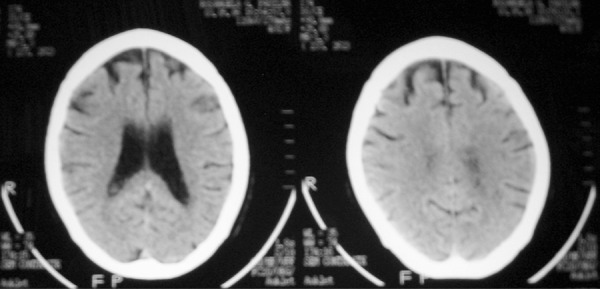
Brain CT showing frontal atrophy.
